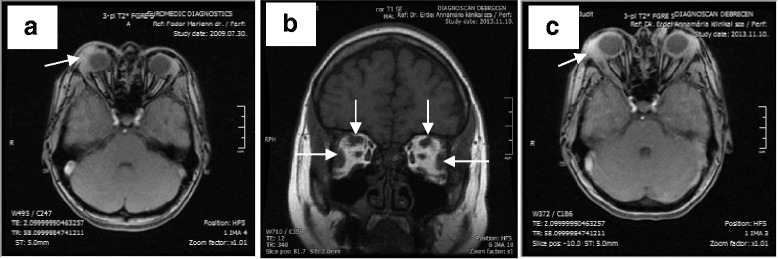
MRI of the orbits. a: T2 axial scan (in 2009). Inflammation in the right lacrimal gland and in surrounding connective tissue (arrow). b: T1 coronal scan (in 2013). The lateral and superior rectus muscles are enlarged in both orbits (arrows). c: T2 axial scan (in 2013). The arrow points to swollen lacrimal gland in the right orbit
